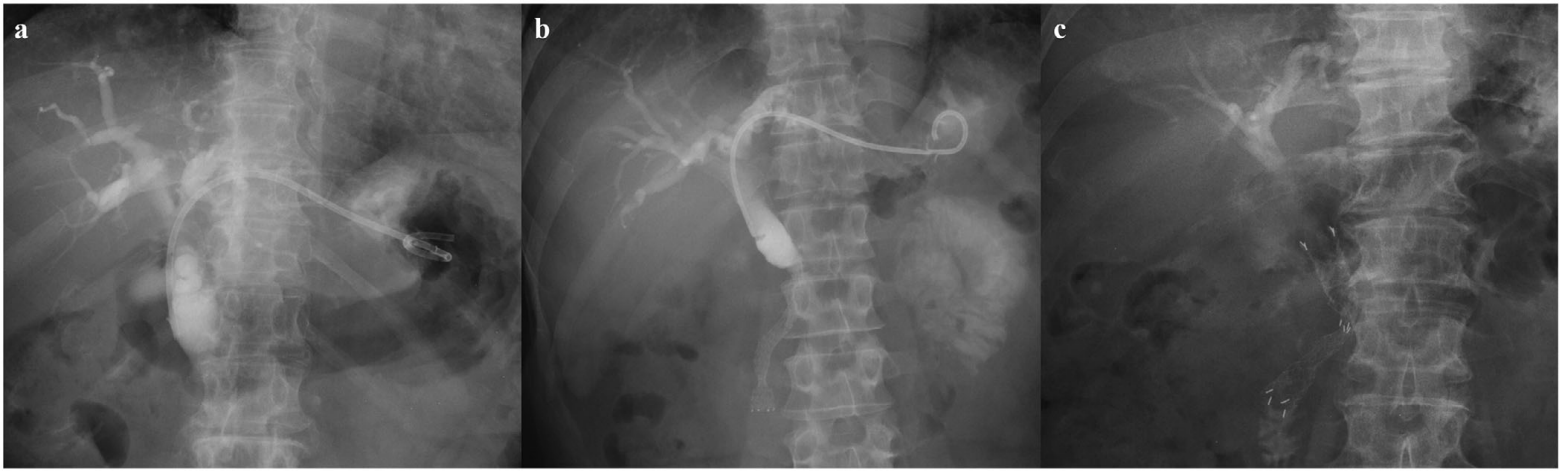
Fluoroscopic X‐ray imaging of endoscopic biliary stenting for malignant distal biliary obstructions. (a) HGS‐PS. (b) HGS‐PS with AS‐MS. (c) EBS‐MS. HGS‐PS, endoscopic ultrasound‐guided hepaticogastrostomy with a plastic stent; AS‐MS, endoscopic ultrasound‐guided antegrade stenting with a self‐expandable metallic stent; EBS‐MS, endoscopic retrograde cholangiopancreatography‐guided biliary stenting with a self‐expandable metallic stent.
